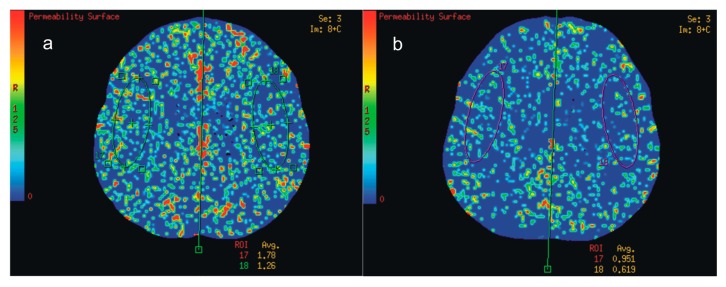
CT (Computed tomography) perfusion colour maps. Permeability surface product area (PS). Regions of interest (ROIs) placed on MCA (Middle cerebral artery) territories. (a) Before right internal carotid artery surgery and (b) after surgery. Decline in average PS after surgery was visible in both hemispheres. Regions of interest (ROIs) placed on middle cerebral artery territories (green and violet ovals on images).
